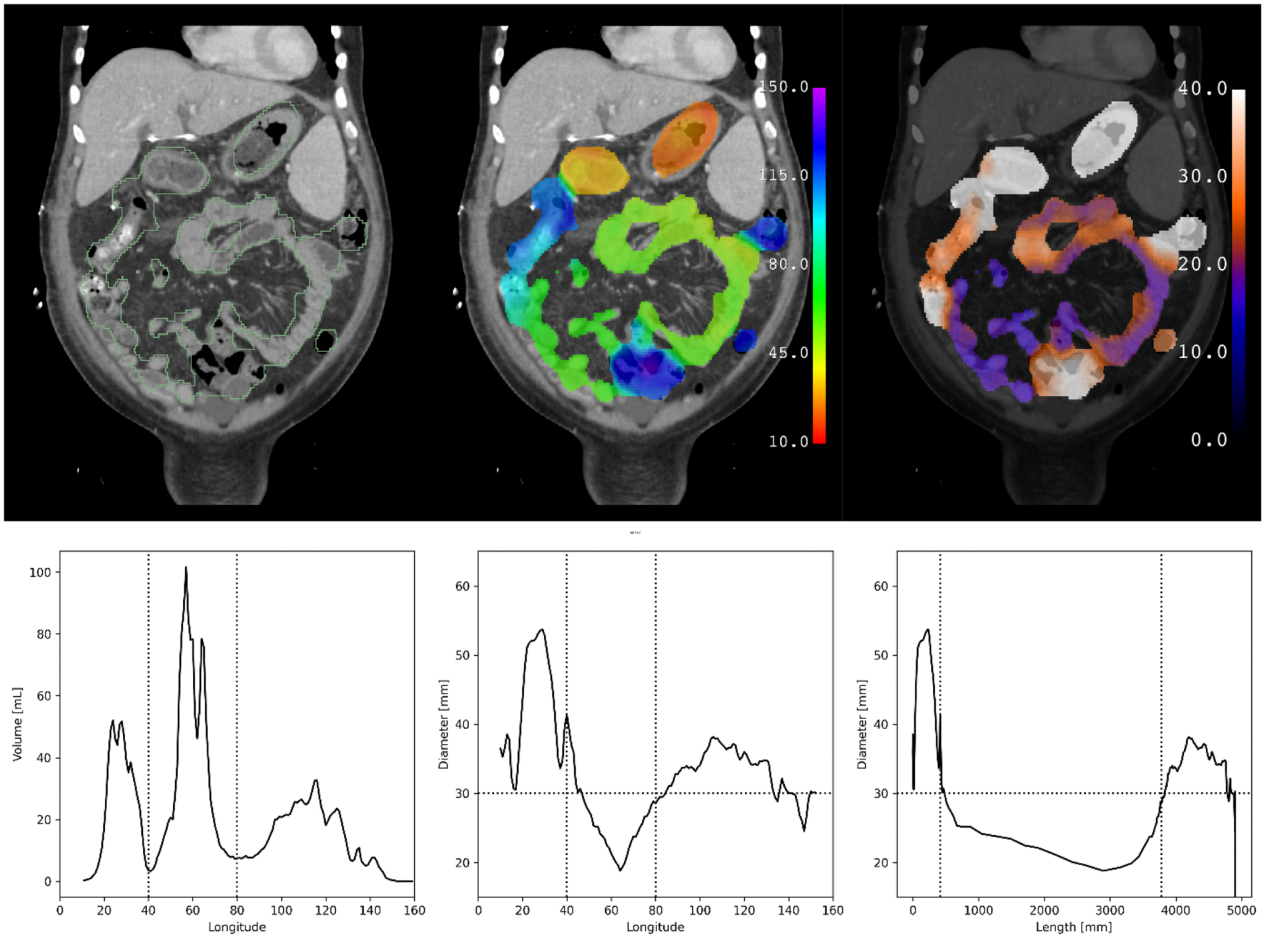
Test subject with no bowel obstruction. Segmentation (green outline), longitude (rainbow), and diameter (hot metal blue) predictions superimposed on a coronal slice of a CT scan of a subject from the test data set with no bowel obstruction. Graphical representations of volume and diameter (averaged over 1 unit intervals of longitude) versus longitude and length are shown for the same subject. Vertical dotted lines indicate the ligament of Treitz and ileocecal valve. Horizontal dotted line indicates the threshold for small bowel obstruction. No small bowel dilation and no transition point are present
